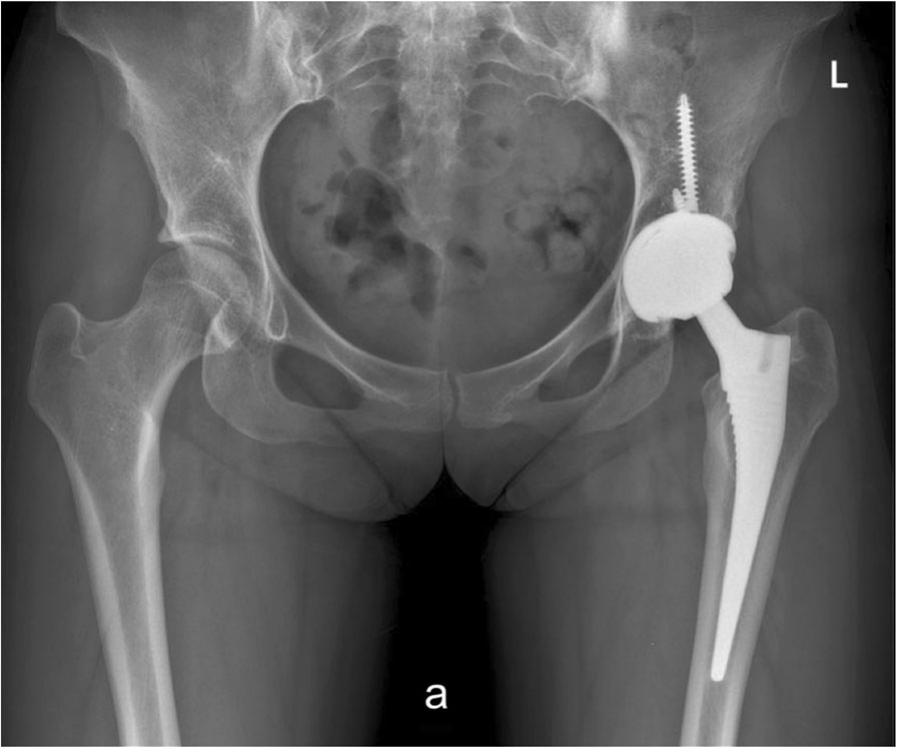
A radiograph was taken 9.1 years after THA showed well-fixed prosthesis (case 16)
